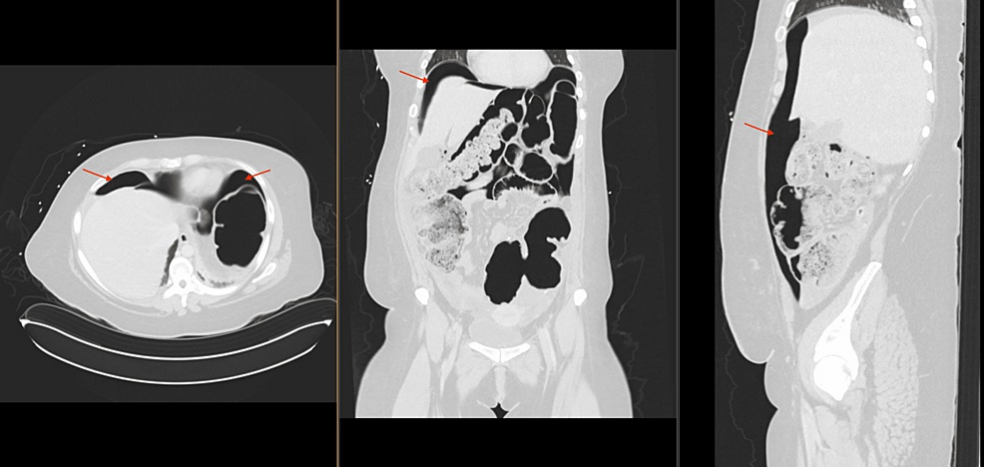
CT images postoperative day eight Large volume free air anastomotic leak (red arrows).
